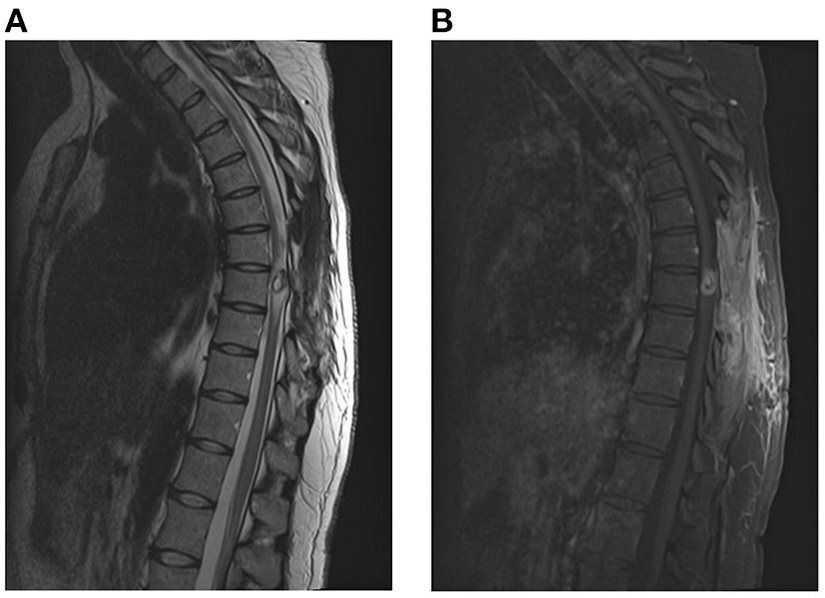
Post-operative MRI with and without contrast of the spine [(A): T2, (B): T1 w contrast], demonstrating interval decrease in size of the lesion as well as decrease in the edema surrounding the lesion.
